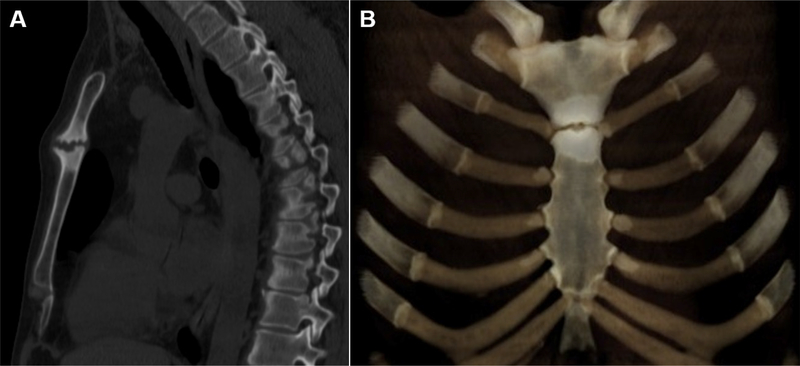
(A) Sagittal CT image (bone window) with (B) 3-D reconstruction demonstrates poorly-defined osteolytic lesion at the sternomanubrial junction with prominent diffused dense sclerosis of the adjacent sternum.
